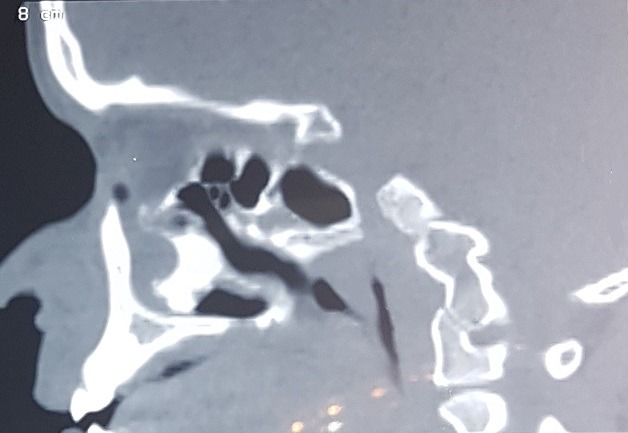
Sagittal sinonasal computed tomography (CT) showing incarceration of the rhinolite at the level of the inferior turbinate
